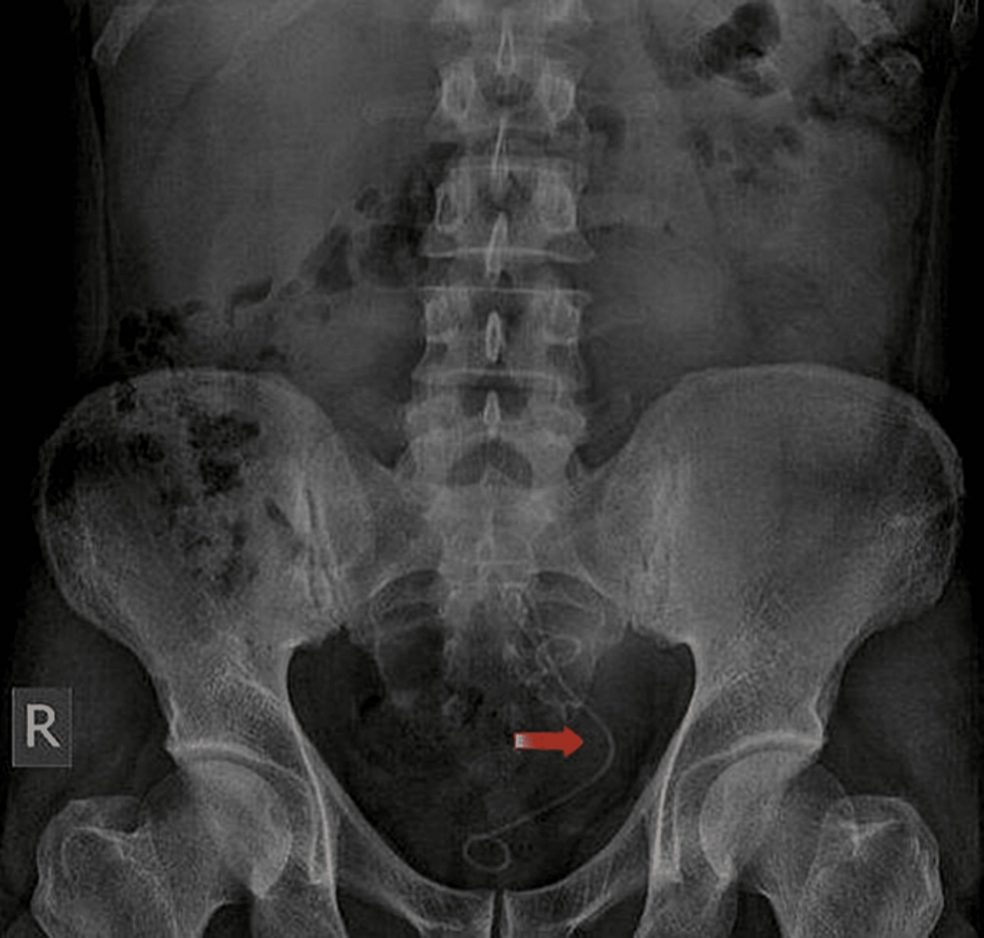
Post-op KUB x-ray showing left double J stent in place with no evidence of residual stone KUB - Kidney Ureter Bladder
